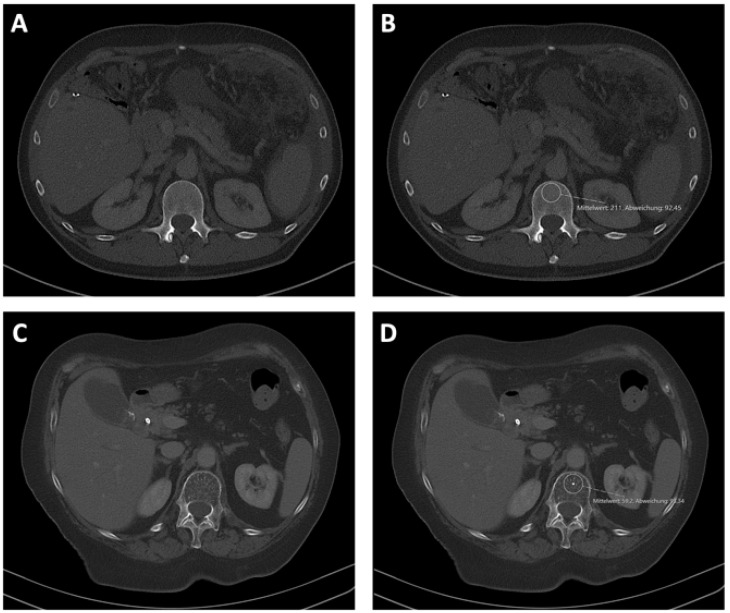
Assessment of BMD at the level of the first lumbar vertebra. BMD was assessed using CT in venous phase and the local PACS (IntelliSpace PACS, Philips, Amsterdam, The Netherlands). Examples of high (A,B) and low (C,D) BMD values with (B,D) or without (A,C) displayed ROI.
